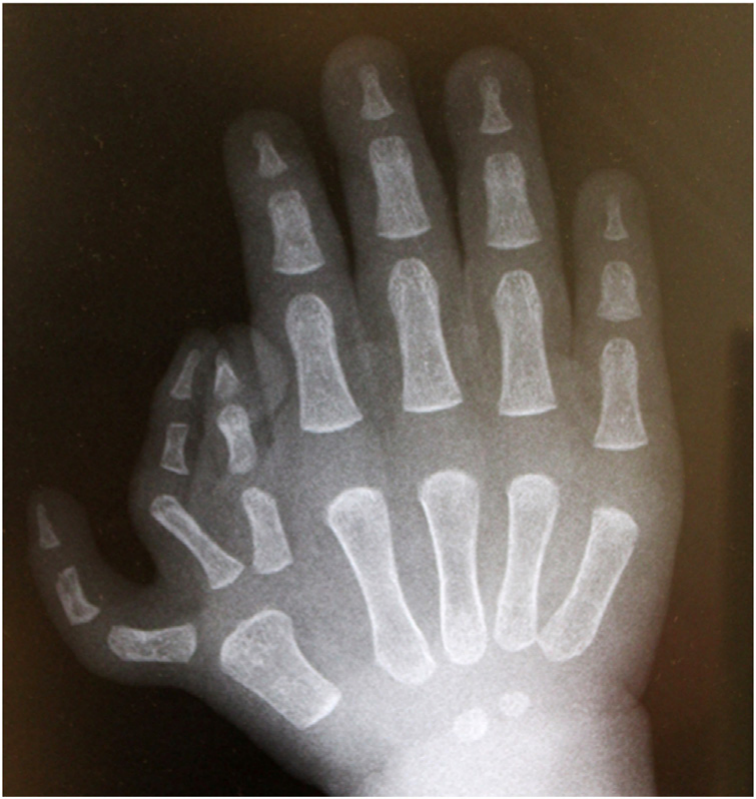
Preoperative radiograph showing triplication, consisting of three triphalangeal thumbs.
